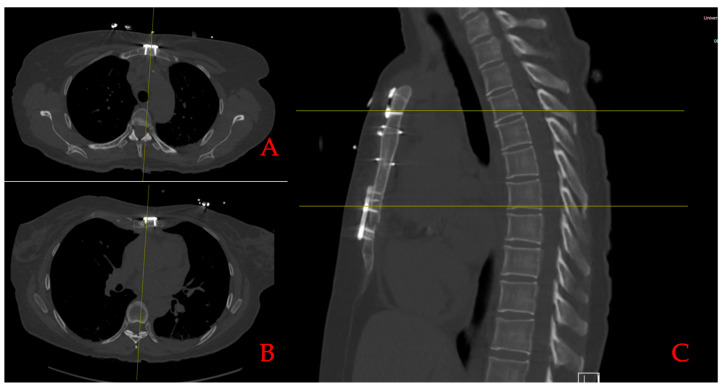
Postoperative CT scan after rigid plate fixation. Postoperative CT scan showing ossification and correct plate position of the rigid plate fixation system at the level of (A,C) the manubrium and (B,C) the corpus sterni.
